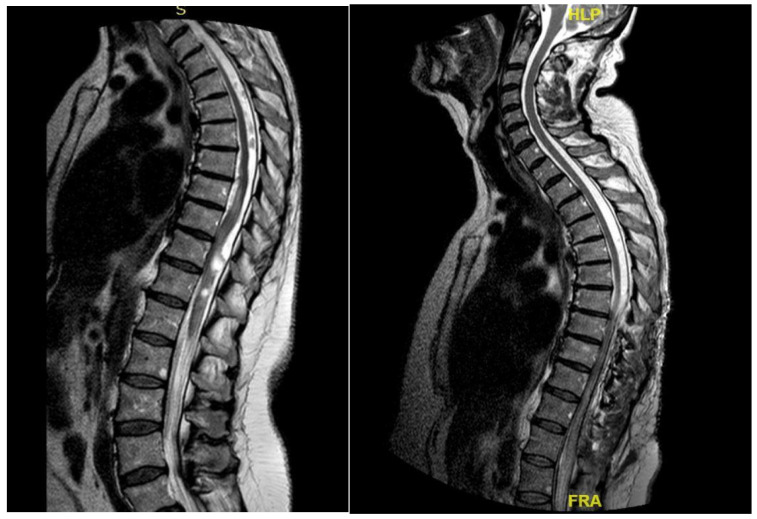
Case 5: pre-operative MRI (left) and post-operative MRI (right).
